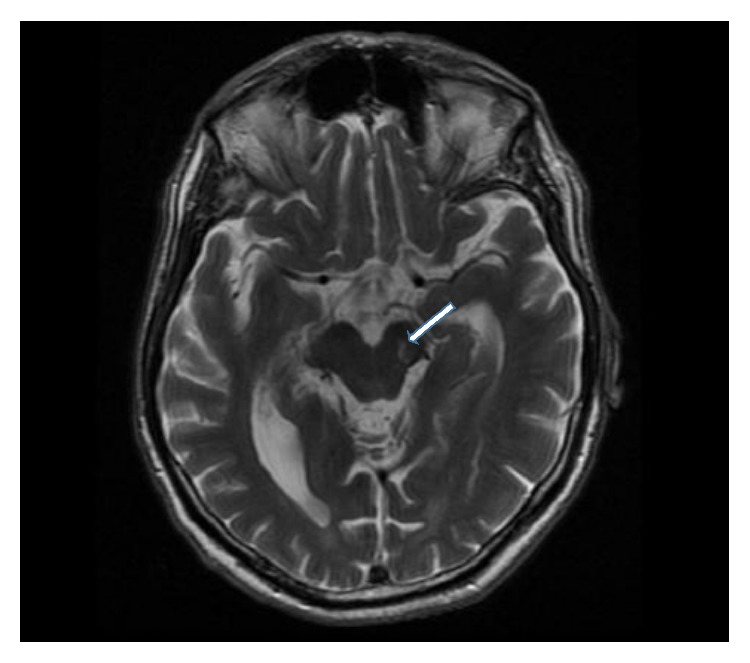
Cranial MRI revealed a lacunar infarction localized in the left midbrain (arrow) appearing hyperintense in T2 sections.
